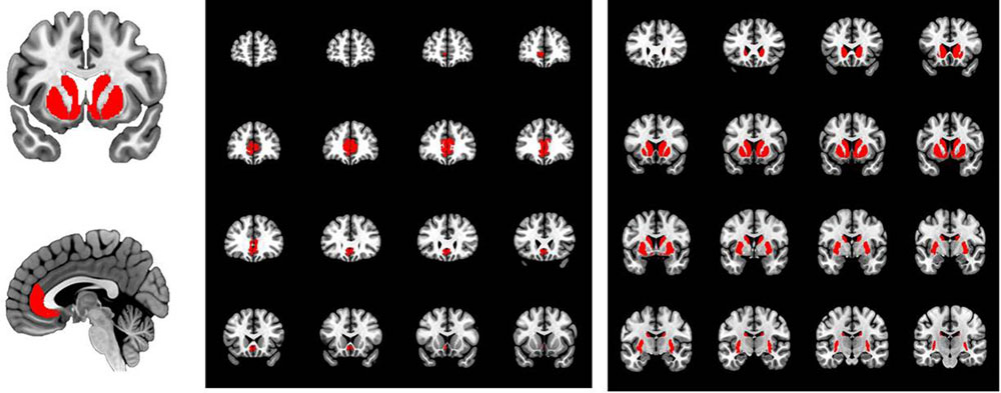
Regions of interest (ROIs) in the striatum and rostral cingulate gyrus as delineated with FreeSurfer software from individual T1-weighted MR images.
